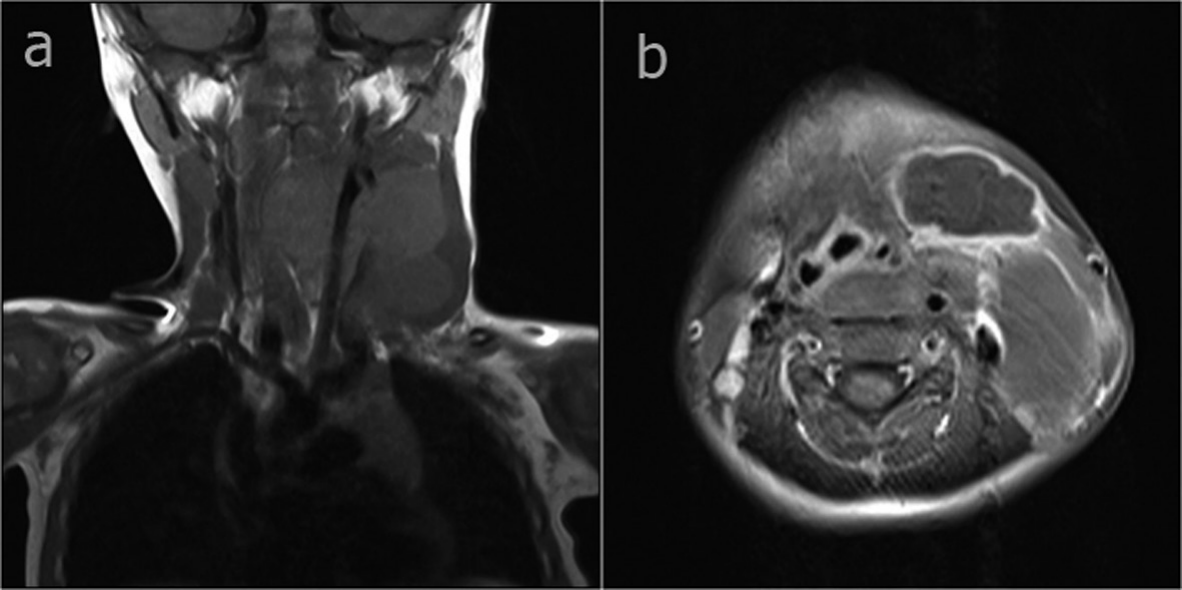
In the coronal T1-weighted image (a) a cystic mass wrapped around the internal jugular vein on intermittent signal dependent on the haemorrhagic and/or proteinous content, extending to the mediastinum from the left cervical region. In the axial fat-suppressed T1-weighted image (b) cystic mass showing contrast observed in the wall of the left cervical region.
